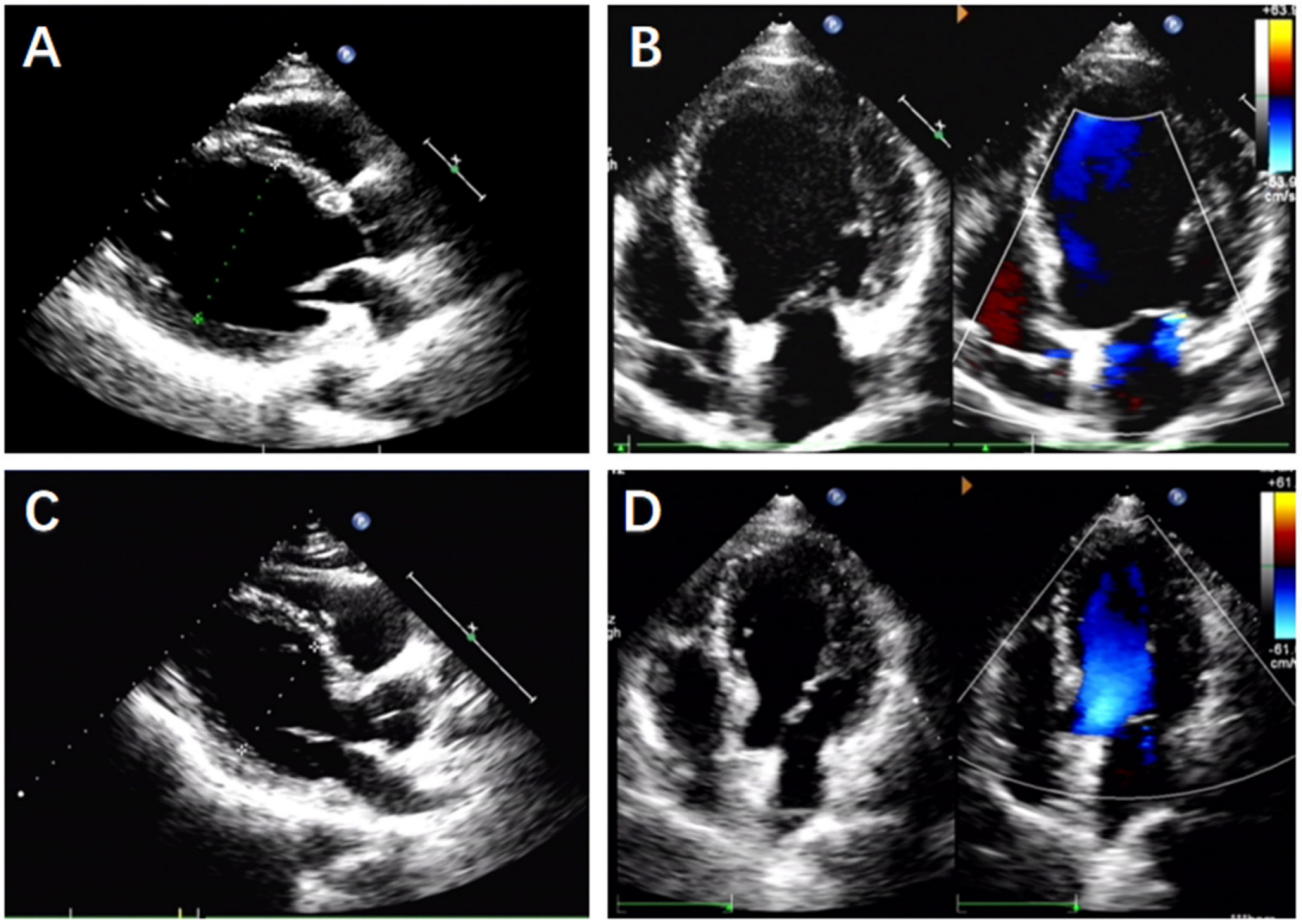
Echocardiogram. (A) In April 2012 chest parasternal view, left ventricular anterior-posterior diameter 7.1 cm. (B) In April 2012 apical five-chamber view. (C) In April 2013 chest parasternal view, left ventricular anterior-posterior diameter 5.4 cm. (D) In April 2013 apical five-chamber view.
